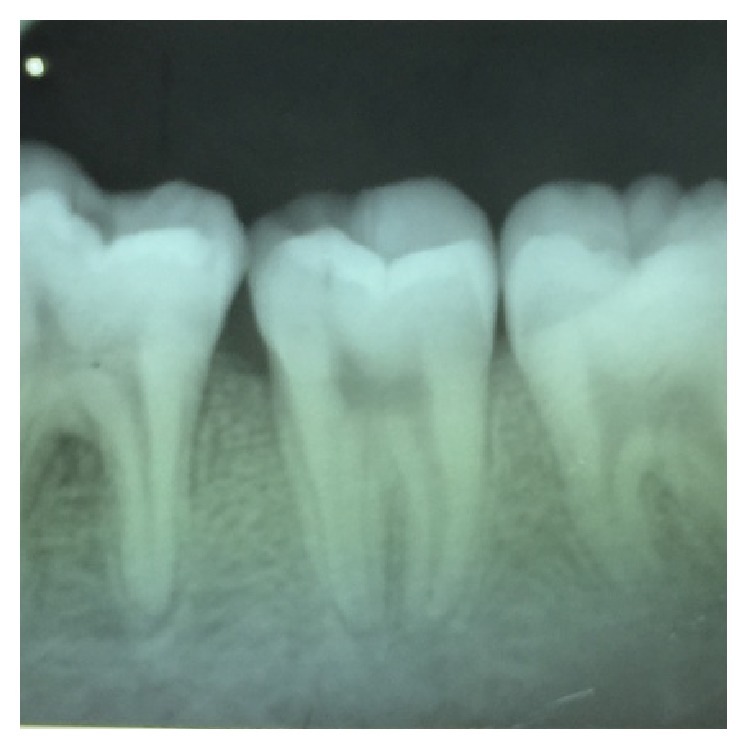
Preoperative radiograph of 37.
